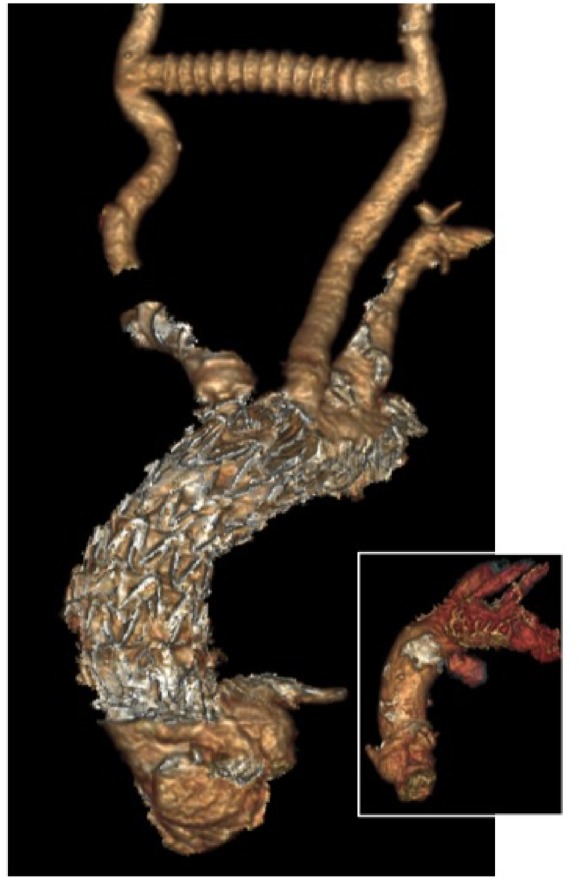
Control computed tomography scan: endovascular stent graft covering the innominate artery in acute aortic plaque rupture.
